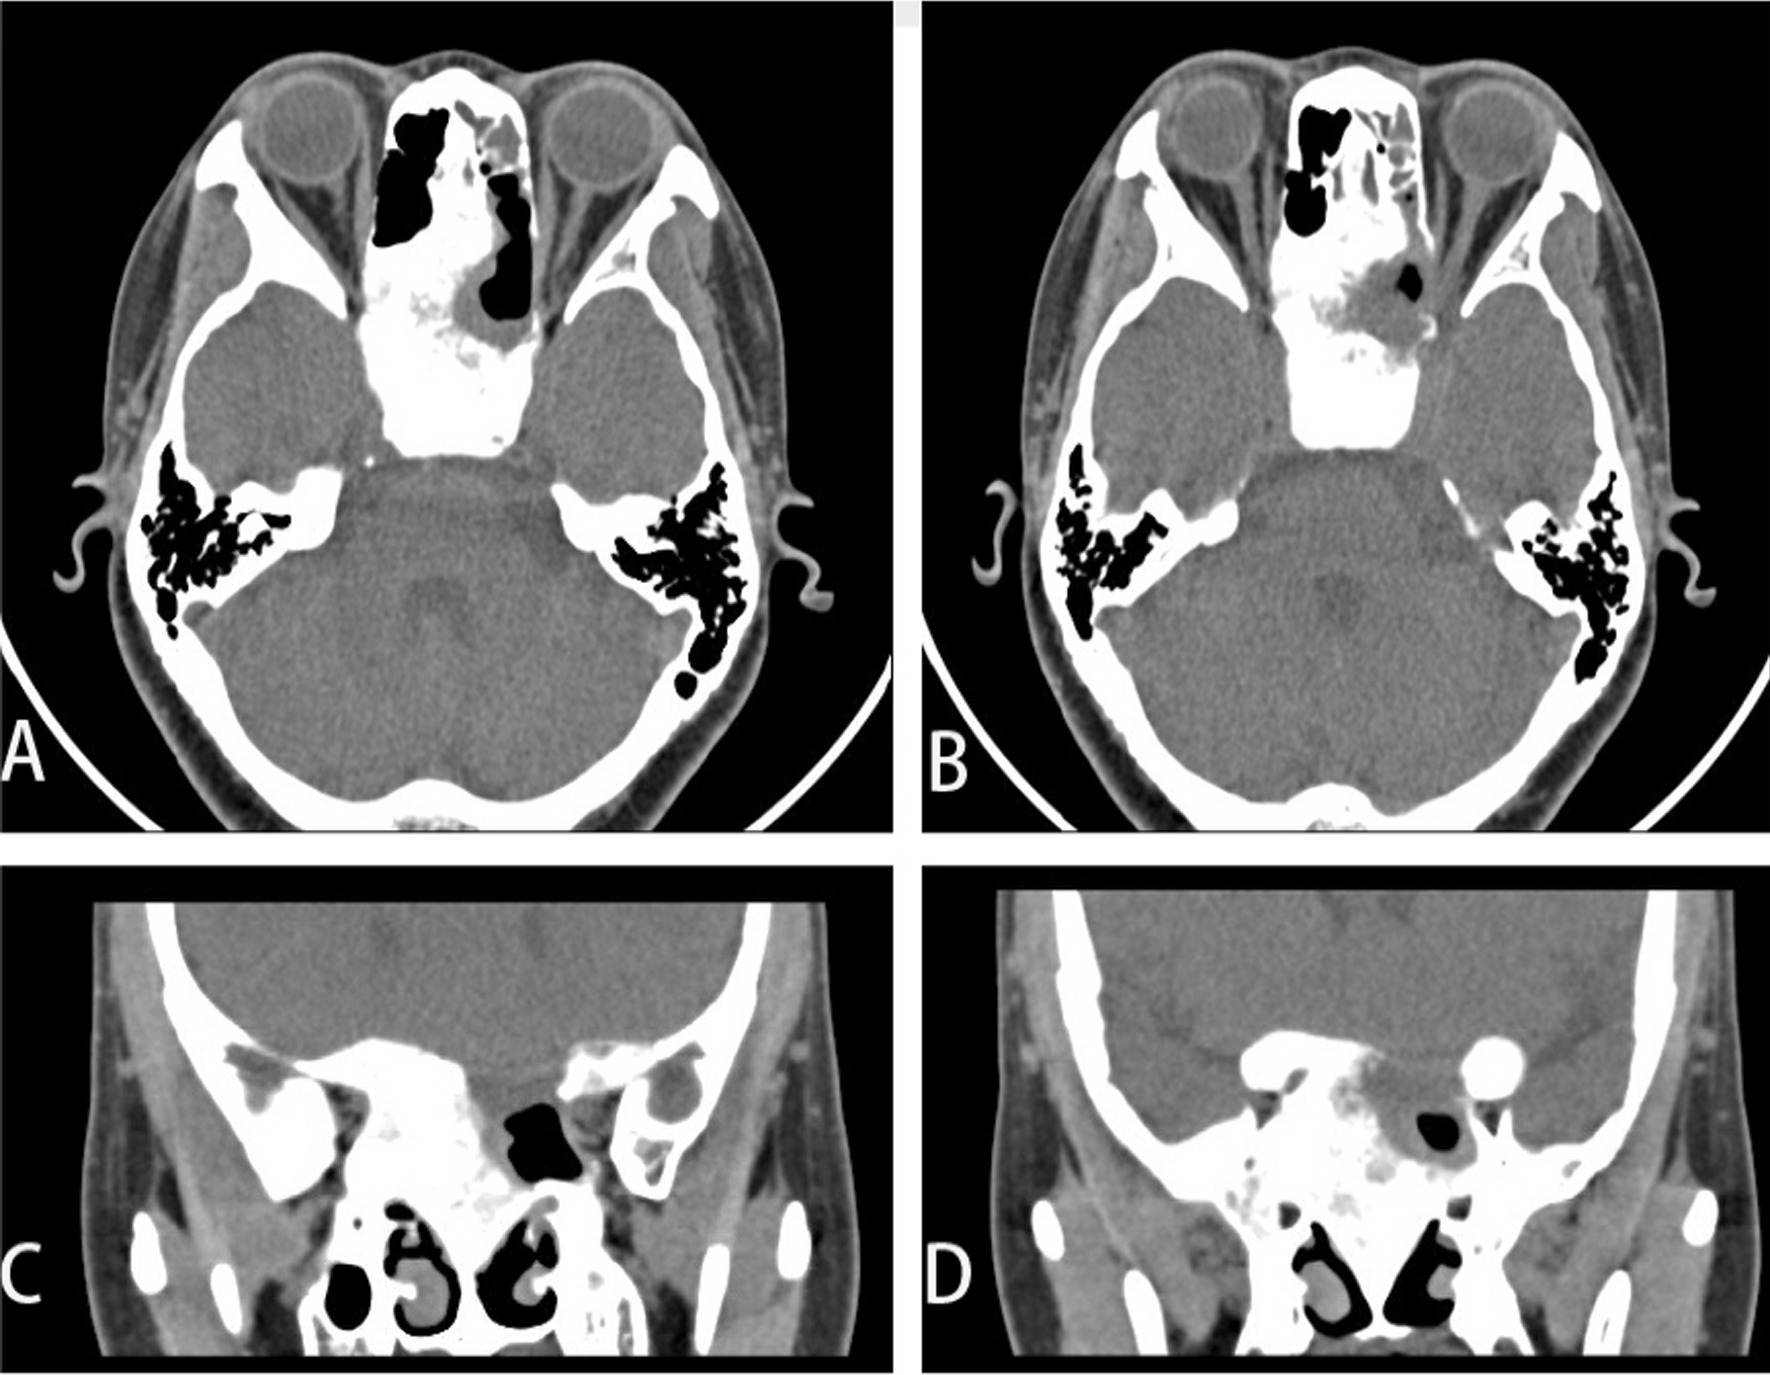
Orbital CT after surgery. A, B, C, D showed that the cystic lesions on the left side were cleared from different angles
